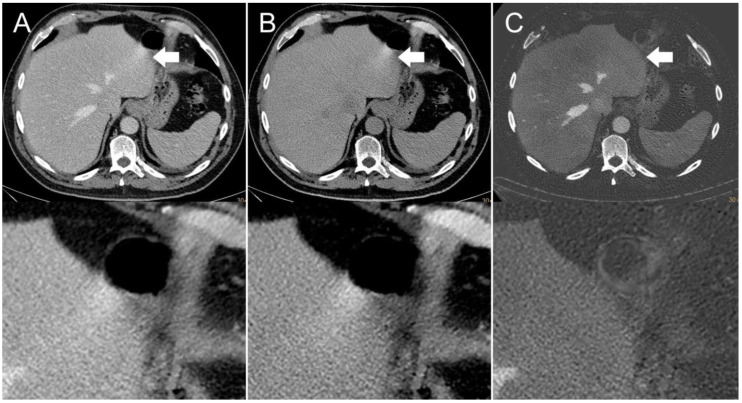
Axial contrast-enhanced CT scan of a 65-year-old man. Streak artifact (indicated by arrows) on the left liver lobe originating from peristaltic motion of the stomach visible in the (A) conventional 120 kVp image and (B) virtual non-contrast (VNC) image reconstruction. The streak artifact is markedly reduced in the (C) iodine image reconstruction, resulting in a better visibility of the underlying liver tissue.
